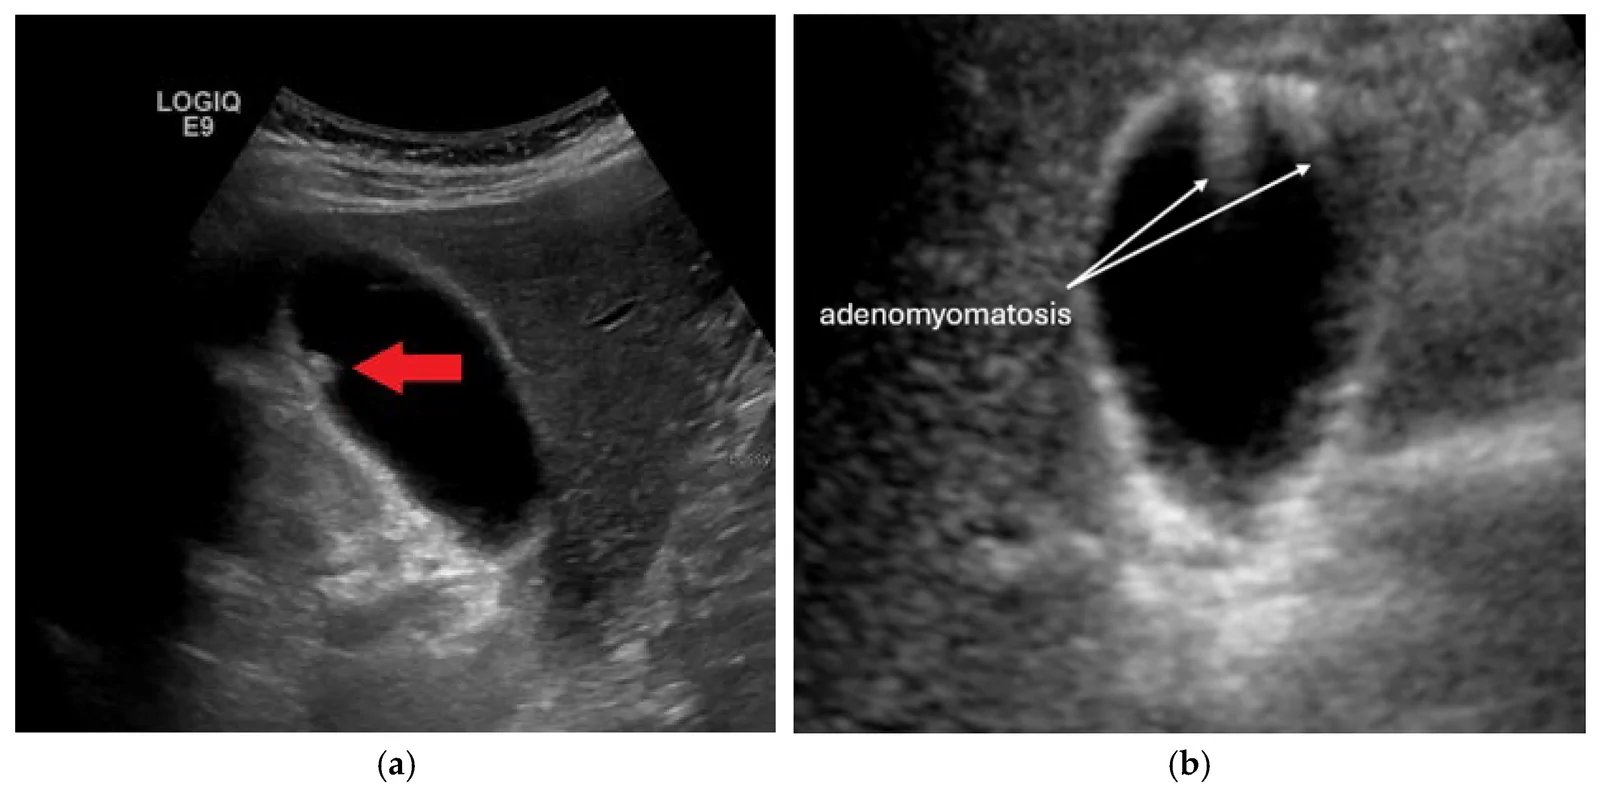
(a,b) While performing POCUS, users should be aware of other non-emergent biliary findings that may be seen. Gallbladder polyps are often asymptomatic and are common incidental findings on abdominal ultrasound. They are divided into pseudopolyps (more common and benign) or true polyps [19,20]. Pseudopolyps include focal adenomyomatoses and cholesterol polyps. True polyps can be benign or malignant and are defined as adenomas or adenocarcinomas [19]. True polyps, as seen in (a), will appear as a small, hyperechoic, non-motile outgrowth of the gallbladder wall that can be pedunculated or sessile, (red arrow) whereas pseudopolyps will display a “comet-tail” artifact [21]. Clinicians should perform a dynamic POCUS assessment to differentiate between biliary sludge and polyps, as sludge is motile and will mobilize off the gallbladder wall when the patient rotates from supine to the LLD position [8,20]. Adenomyomatosis is a type of pseudopolyp that is often benign and characterized by excessive growth of the gallbladder wall that can progress into mural diverticula known as Rokitansky–Aschoff sinuses (RAS). These formations appear as intramural anechoic spaces on POCUS and when laden with calculi or cholesterol crystals generate a “comet-tail” artifact (b). This is due to the ultrasound beam generating reverberation artifact off the aggregates within the RAS [8,20,22,23]. The comet-tail artifact is typical but not unique to adenomyomatosis, as it is also seen in cholesterol polyps [19,22,23]. Patients with benign findings typically do not require acute intervention and can follow up for surveillance or further outpatient imaging with a general surgery referral.
